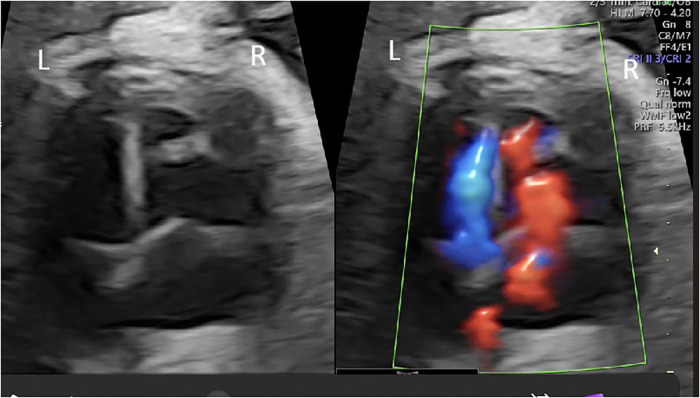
Simultaneous B-mode and color Doppler ultrasound image of the first case. In addition to the mitral and tricuspid valves, a more remarkable structural formation within the right ventricle and inflow toward the most distal region can be seen with color Doppler.
